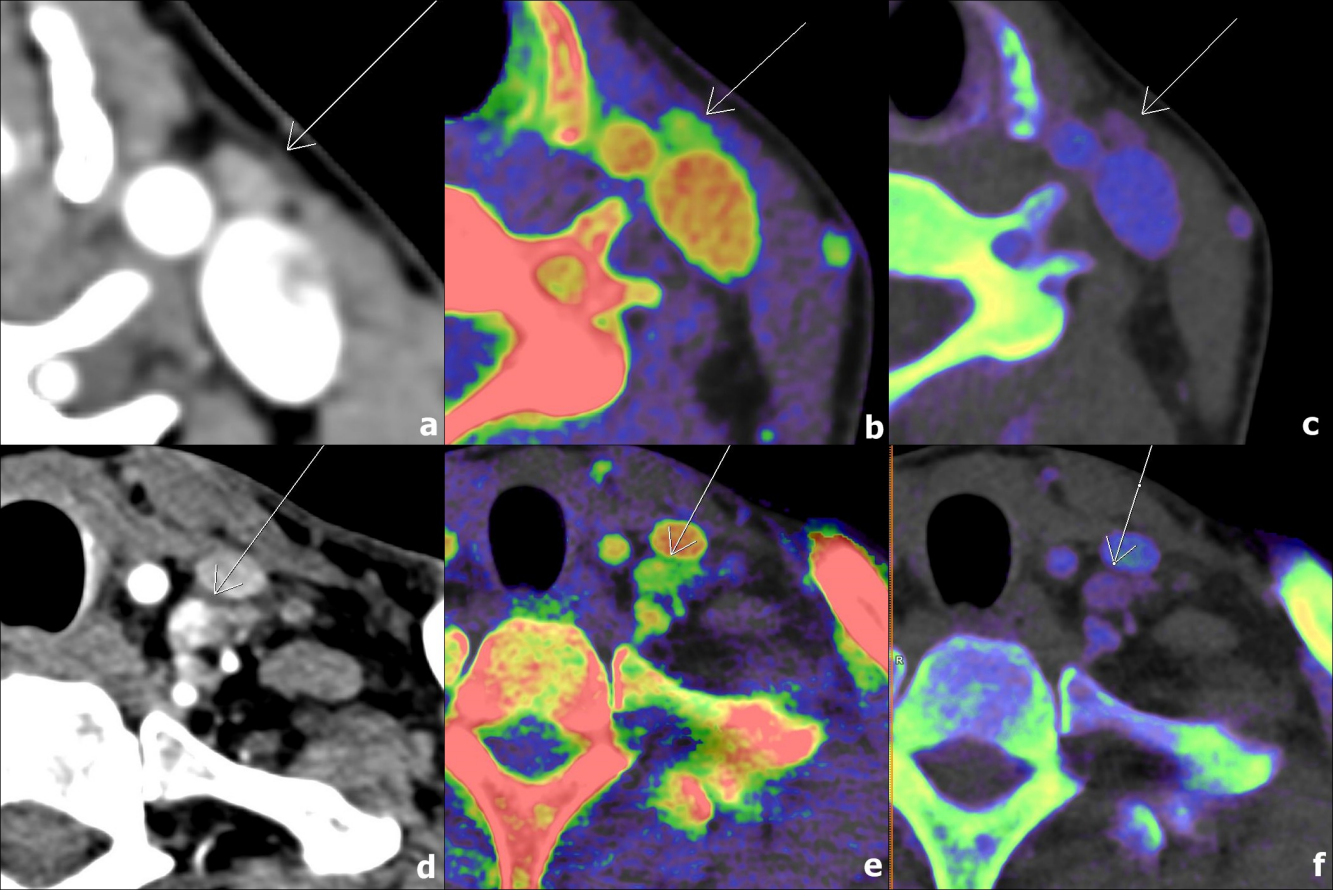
Axial CT images of arterial phase (a, d), DECT-derived contrast-enhancement (b, e), and Rho/Z (c, f) maps of two patients with papillary thyroid carcinoma. A 47-year-old female patient with a benign lymph node at left cervical level II (a, b, c; AUC_100keV_ = 36.7, HU_arterial_ = 0.32, Z_eff_ = 0.91, CM = 0.58, IC = 0.56, λ_HU_ = 4.7), and a 53-year-old male patient with a metastatic lymph node at left cervical level IV (d, e, f; AUC_100keV_ = 43.5, HU_arterial_ = 0.41, Z_eff_ = 0.93, CM = 0.62, IC = 0.58, λ_HU_ = 5.6).
